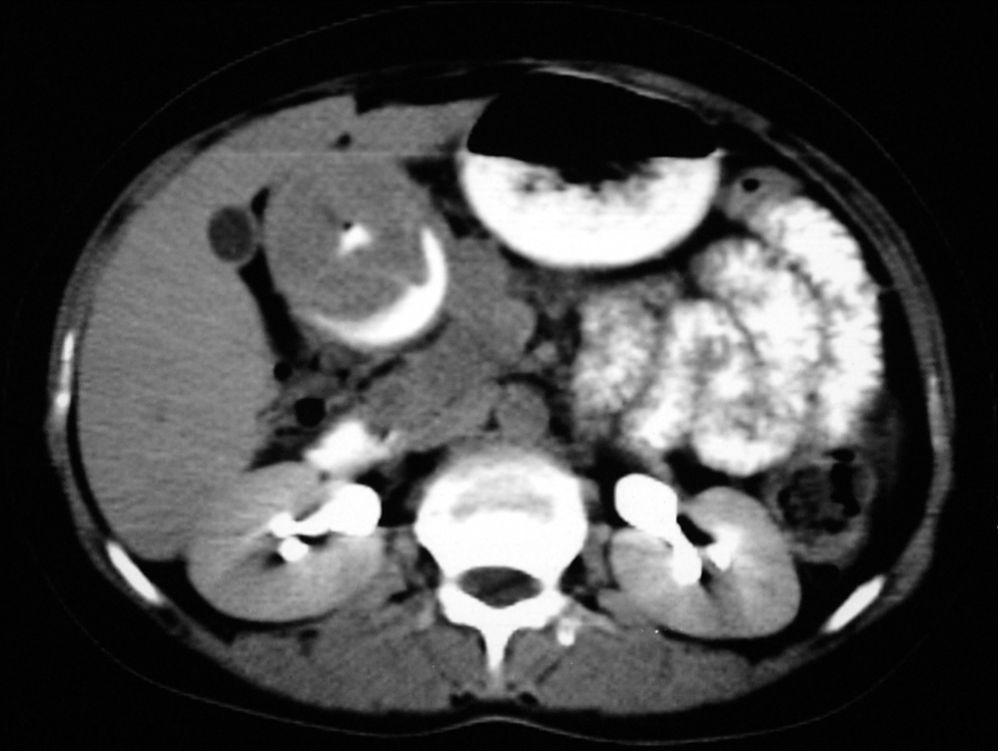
Abdominal CT scan: axial view showing an 8 cm in size antral intramural mass.
